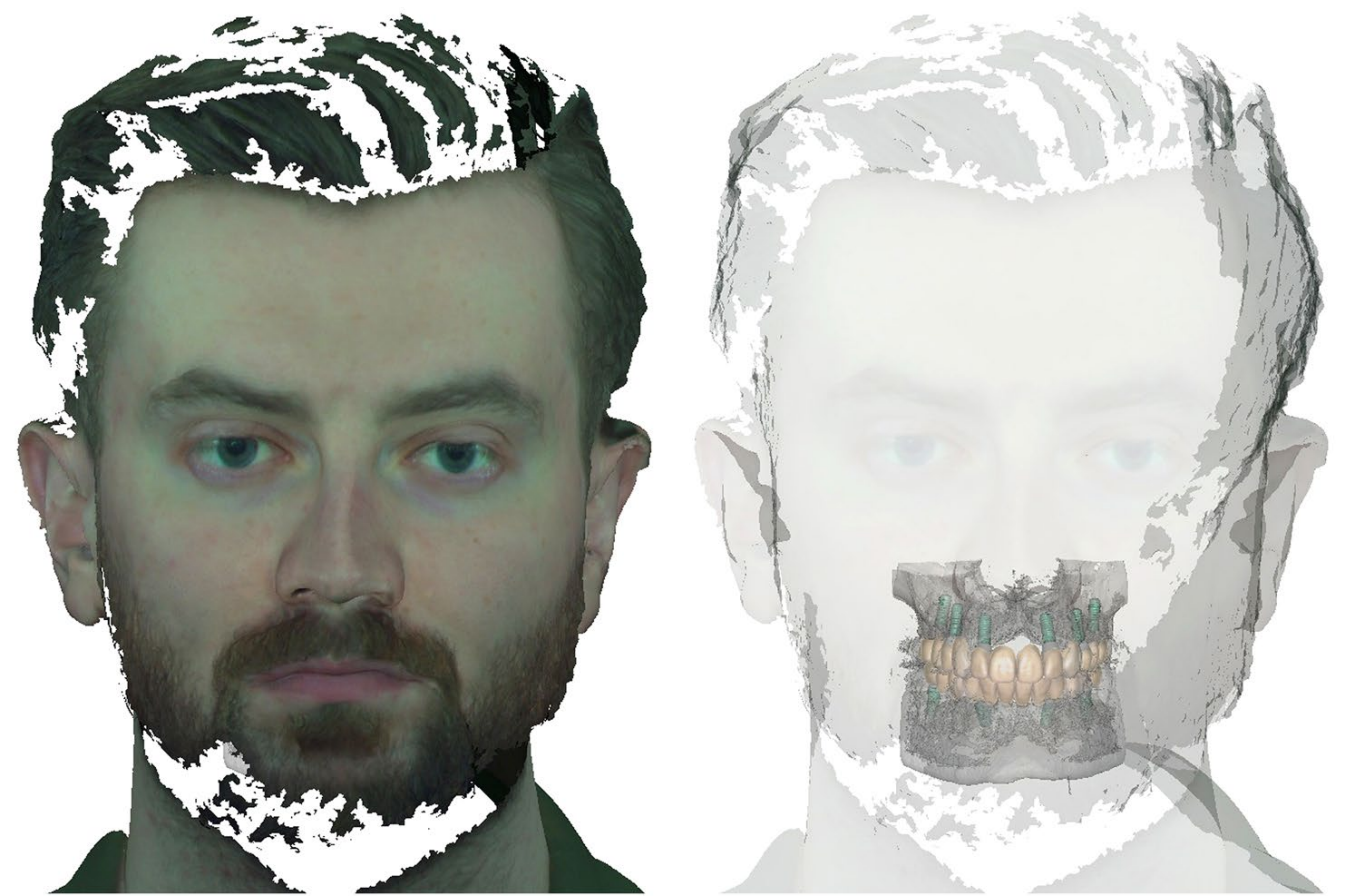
A digital twin of the patient comprising intraoral surface data, radiographic data, and a face scan, enabling comprehensive prosthodontic and implant pre‐planning (DentalCAD, 3.2 Elefsina; Exocad, Darmstadt, Germany), including functional and esthetic aspects, in a patient with early tooth loss due to a rare genetic disease (Informed consent was obtained from the patient).
